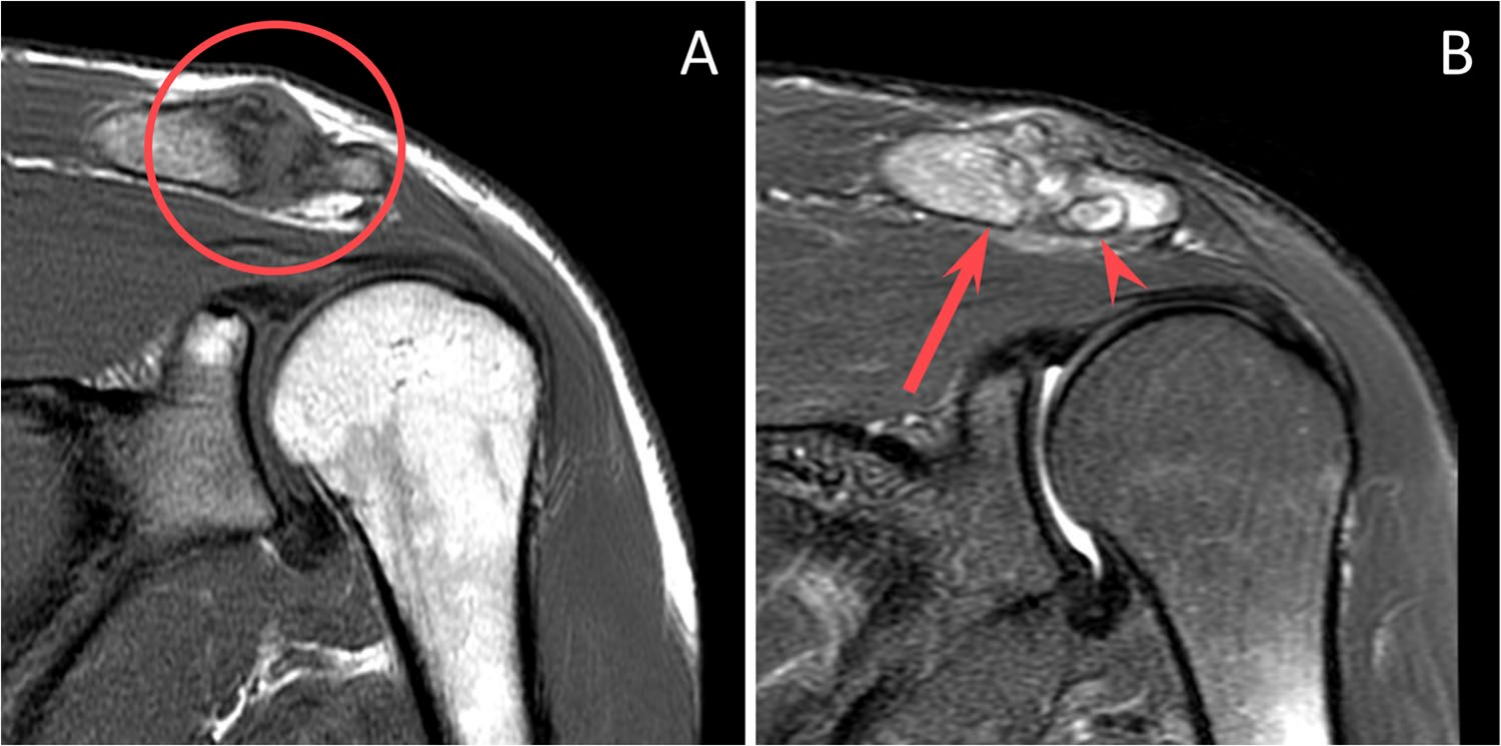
A 32-year-old weightlifter with ongoing shoulder pain for 8–9 months. Coronal T1 (A) MR image demonstrates osteolysis spanning the acromioclavicular joint, with bone resorption, loss of cortical margins, and T1-hypointensity (circled). Coronal STIR (B) MR image demonstrates intense bone marrow edema with osseous resorption and cystic change in the distal clavicle (arrow) and anterior acromion (arrowhead). Note that there is a suggestion of mild underlying acromioclavicular joint osteoarthritis, with small marginal osteophyte formation at the superior margin of the joint
